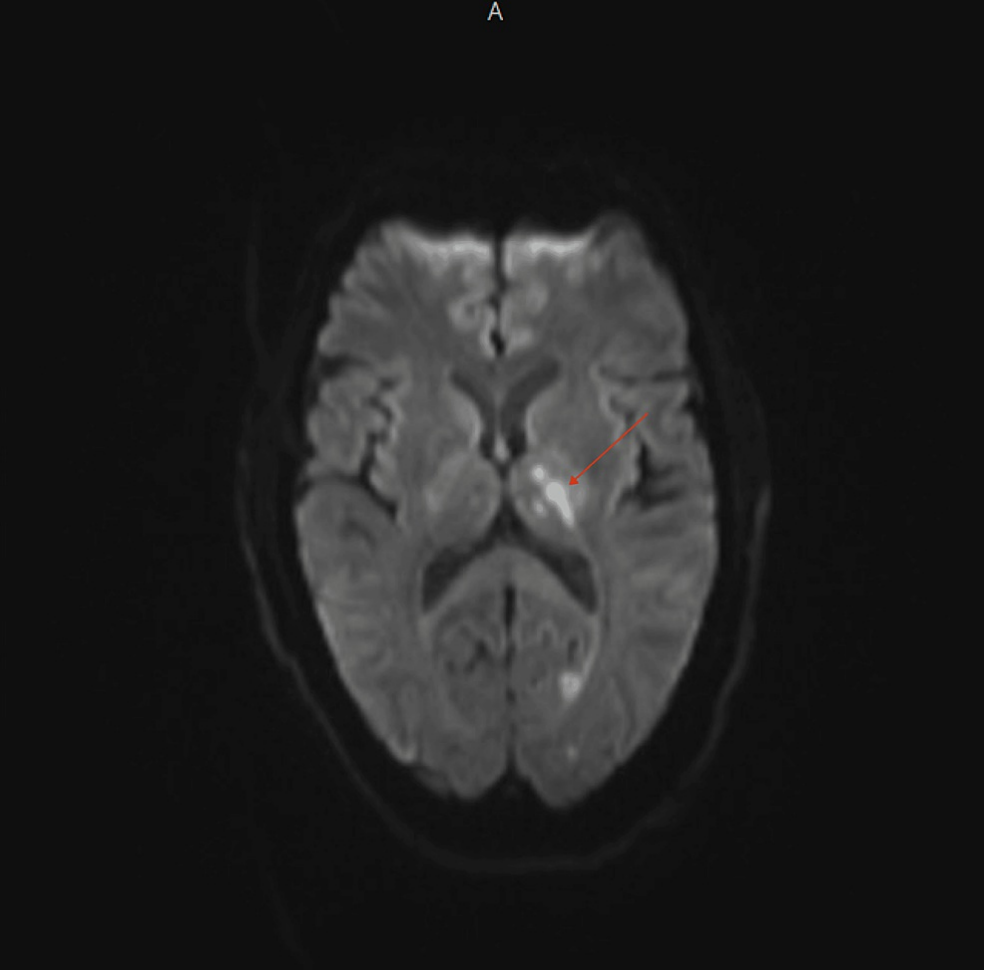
Diffusion-weighted MRI of the brain showing an area of diffusion restriction at the junction of the left thalamus and posterior limb of the internal capsule
